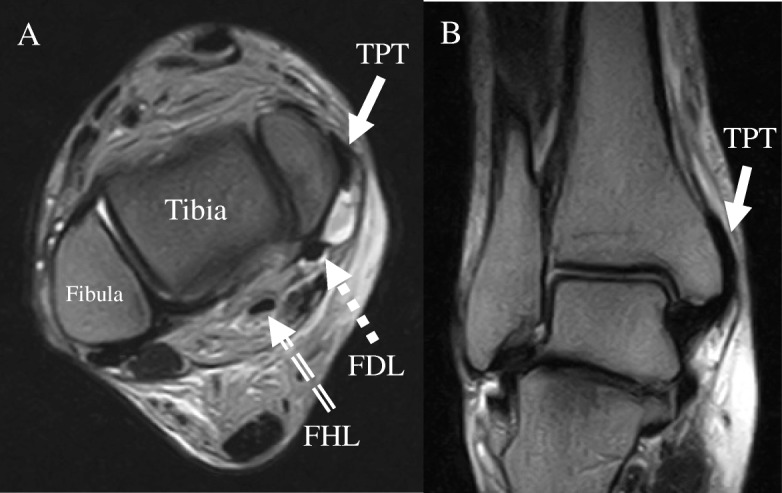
Magnetic resonance imaging demonstrates an anterior subluxated tibialis posterior tendon on the medial malleolus. Effusion is present at the retromalleolar groove. a T2-weighted MRI scan in the transverse plane. b T2-weighted MRI scan in the coronal plane. TPT (tibialis posterior tendon), FDL (flexor digitorum longus tendon), FHL (flexor hallucis longus tendon)
